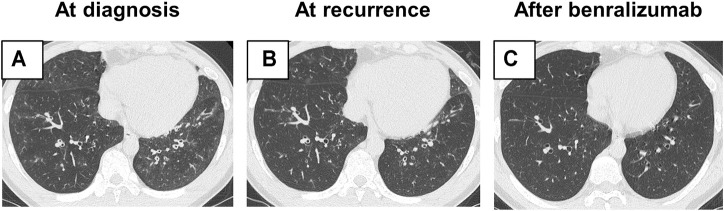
Radiological findings. (A) High-resolution computed tomography (HRCT) findings on admission showing bronchial wall thickening and centrilobular nodules in the lower lobe of both lungs. (B) At recurrence, HRCT showed radiological findings similar to those seen on admission. (C) After the addition of benralizumab, bronchial wall thickening and centrilobular nodules improved.
